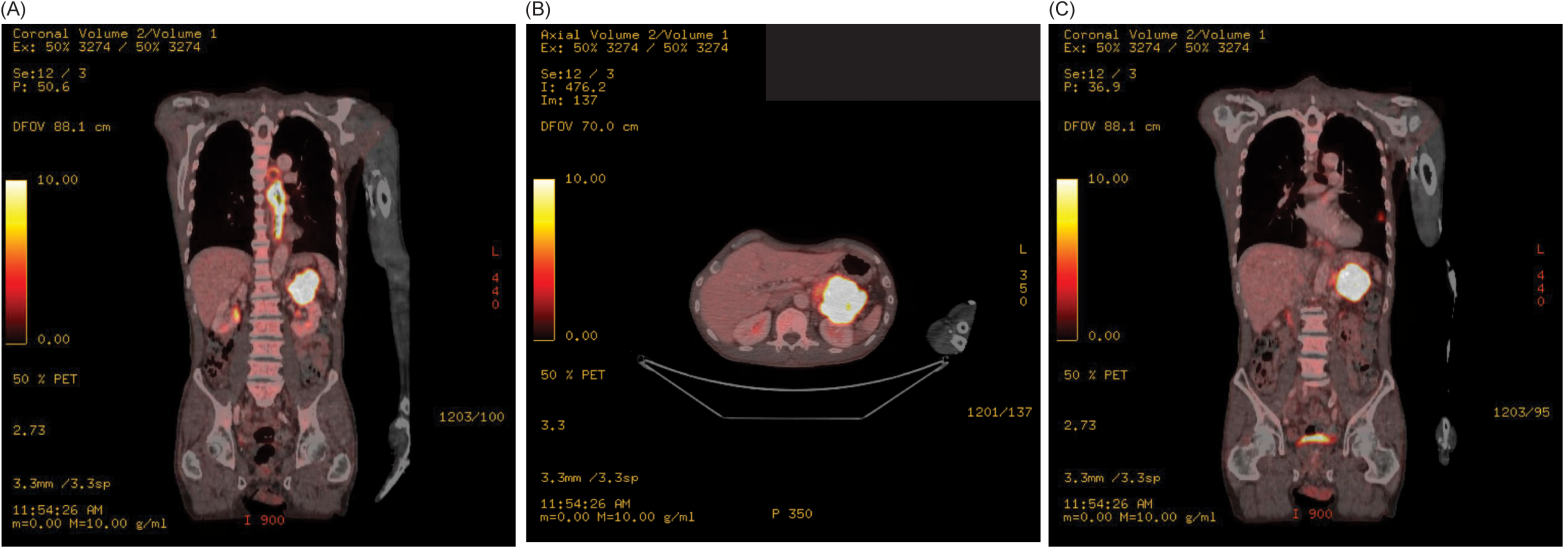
(A) Staging Positron Emission Tomography/Computed Tomography scan demonstrates a diffusely thickened esophagus with FDG avidity in the upper esophagus at the level of the aortic arch to the gastroesophageal junction, and FDG avid pancreatic tail mass, in the coronal plain. (B) Staging Positron Emission Tomography/Computed Tomography demonstrates FDG avid pancreatic tail mass in the axial plane. (C) Staging Positron Emission Tomography/Computed Tomography scan demonstrates a left lower lobe superior segment FDG avid pulmonary nodule in the coronal plain.
